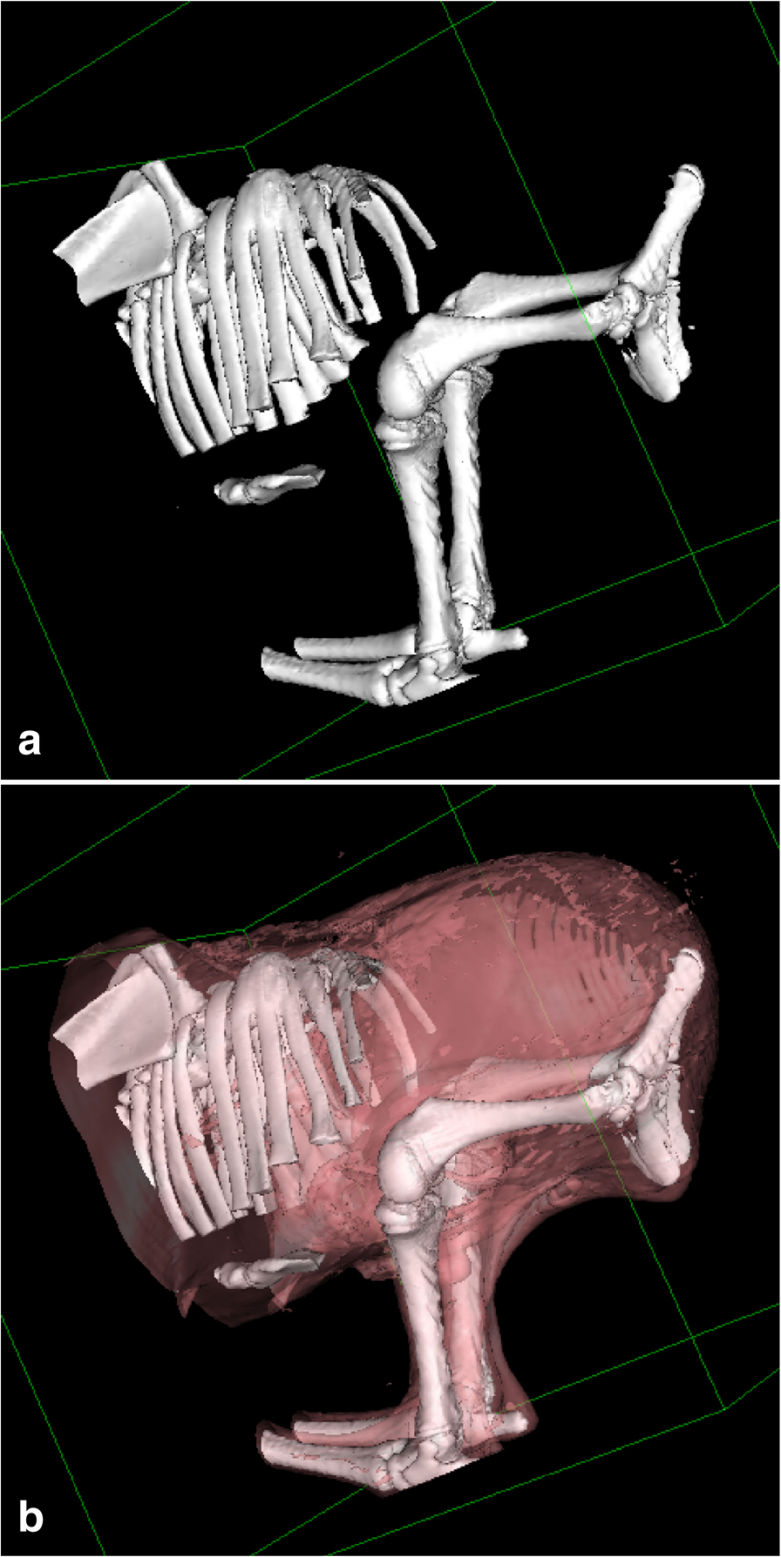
Surface rendered computed tomography images of perosomus elumbis case DK1. a) Surface rendering^a^ to show bone surfaces only. Absence of lumbar, sacral and coccygeal spine can be appreciated. The most posterior thoracic vertebrae are abnormally shaped and the posterior rib pairs are abnormally crowded. b) Same data set as in a), but rendered with bone and soft tissue surfaces. The latter has been set to have a degree of transparency in the reconstruction thus allowing visualisation of the calf’s overall morphology and its relation to the underlying skeletal abnormalities.
